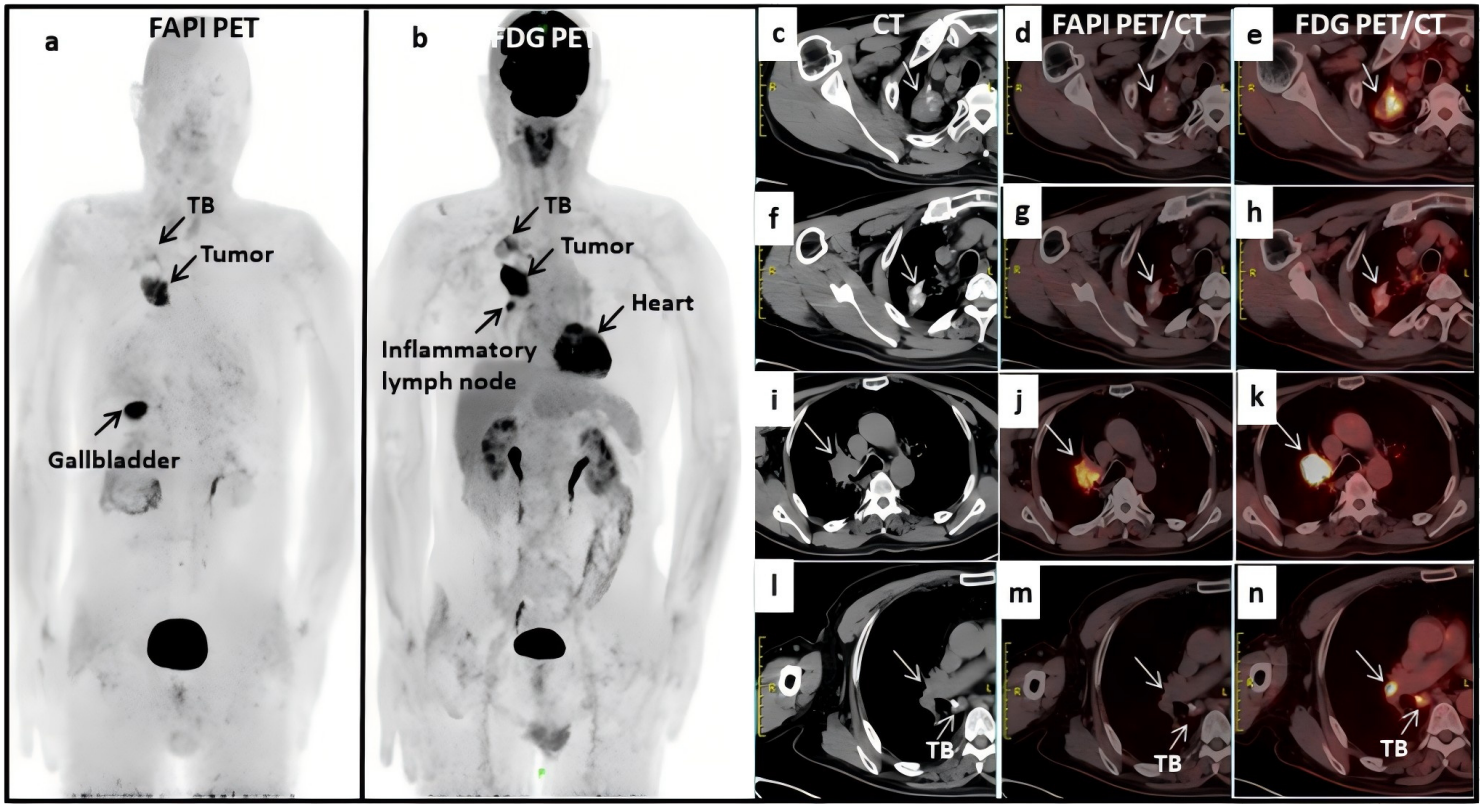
(A, B) full body scans with fluorine 18 [^18^F]- labeled fibroblast activation protein inhibitor (FAPI) PET/CT and [^18^F]-labeled fluorodeoxyglucose (FDG); (C–H) the old calcified focus and scar tissue of the right lung in CT and FDG PET/CT scans, which were considered as TB; (I–K) the metastatic lymph nodes fused with the primary tumor; (L, M) the right hilar lymph node was considered an inflammatory lymph node; (D, G, M) no avid uptake was observed in Al [^18^F]-NOTA-FAPI-04 PET/CT scans.
